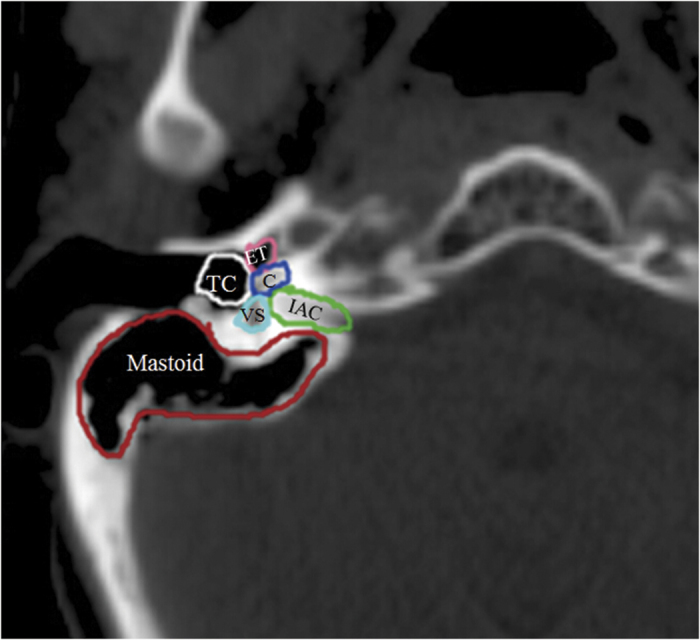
CT imaging of the anatomy of the Eustachian tube (ET), tympanic cavity (TC), mastoid air cells (Mastoid), vestibular apparatus (VS), cochlea (C), and internal auditory canal (IAC).
